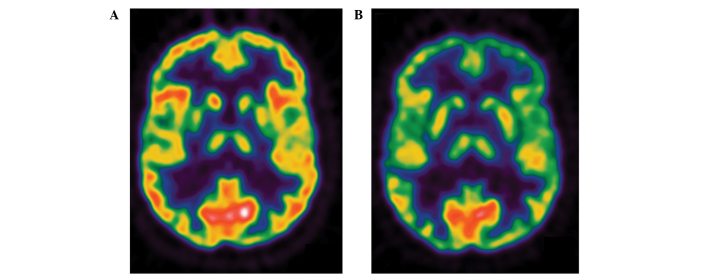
Brain 2-[18F] fluoro-2-deoxy-D-glucose positron emission tomography (PET)/computed tomography scan (A) within one week of Hodgkin disease diagnosis (PET0) and (B) 15±5 days after the first two adriamycin, bleomycin, vinblastine and dacarbazine cycles (PET2).
